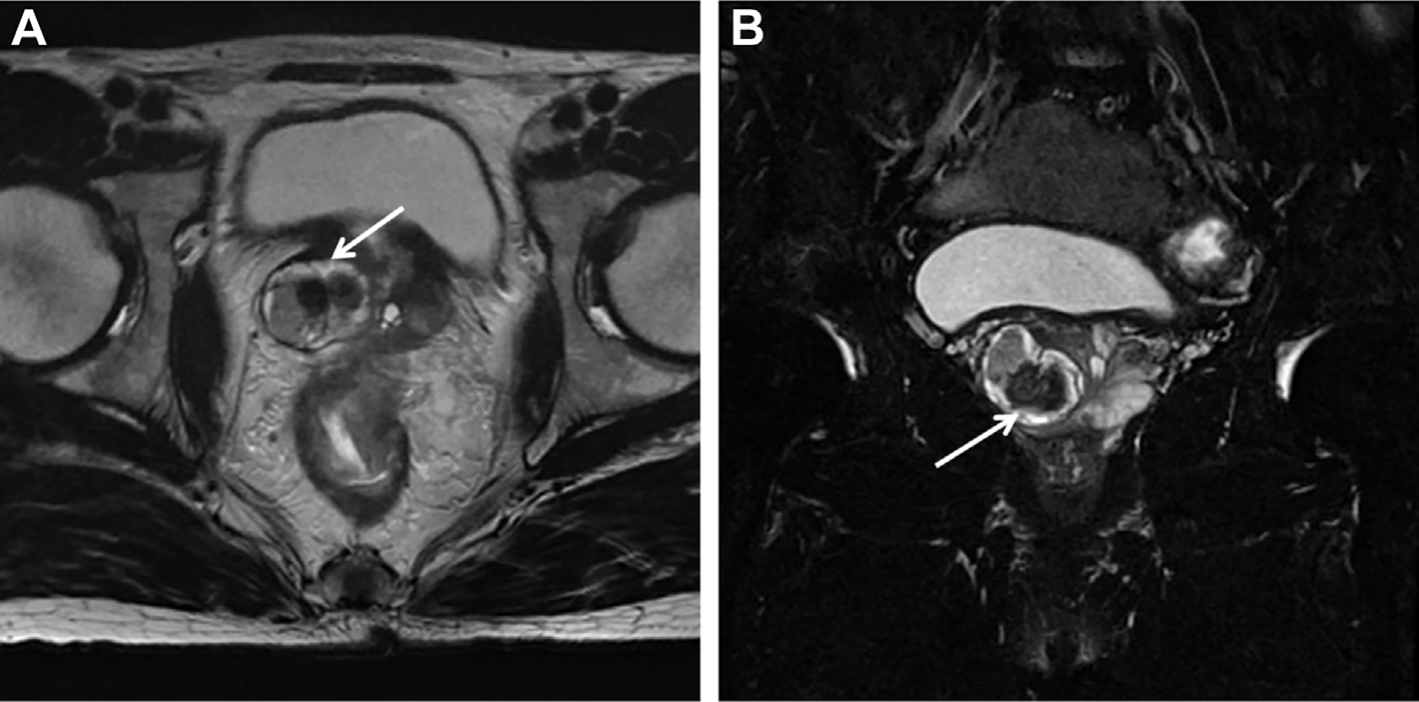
MRI detected a round-like mixed signal lesion in the right lobe of the prostate. T2WI showed mixed signal, with high signal around the periphery and a cluster-like low signal in the center. The lesion boundary was clear, with visible capsule, and the diameter was about 36 mm. The right peripheral zone of the prostate was compressed. (A) Axial T2-weighted Image. (B) Coronal Fat-suppressed T2-weighted Image.
